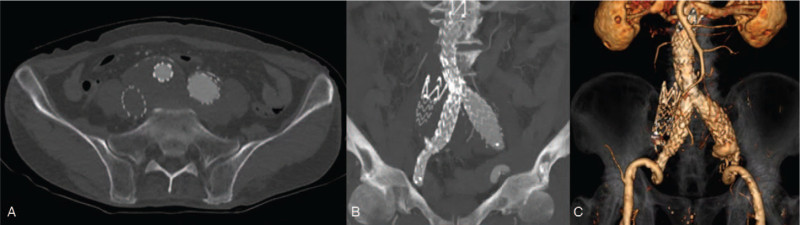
The follow up contrast-enhanced computed tomography at 6 months showed complete resolution of the arteriovenous fistula with no contrast leakage into aneurysm sac and good patency of stent-graft in the axial (A), sagittal (B), and 3D reconstruction (C) views.
